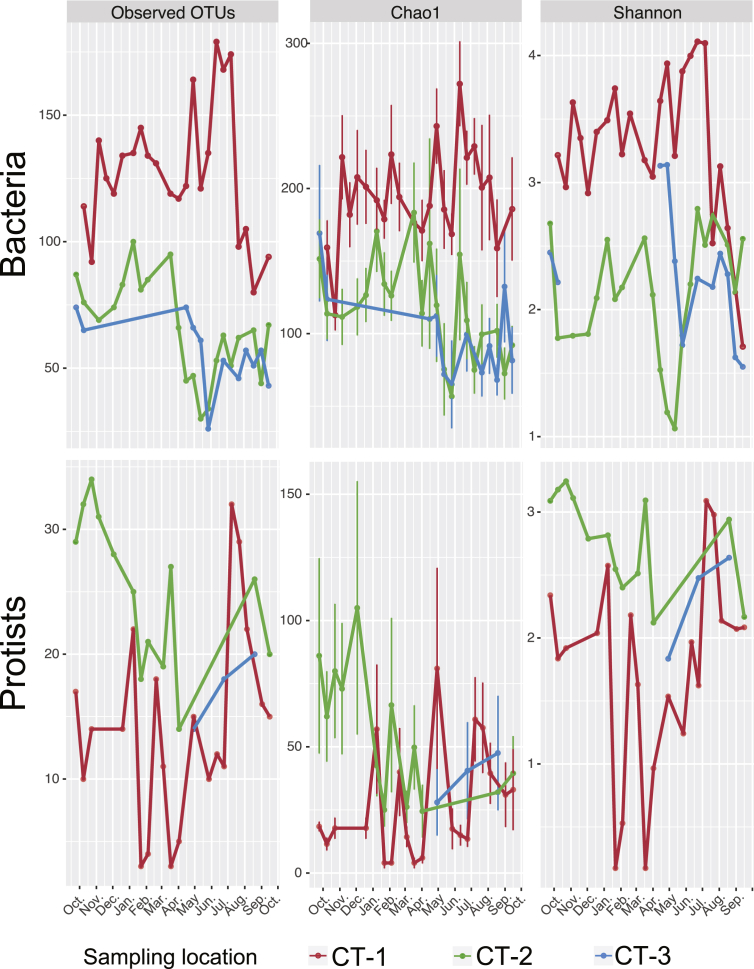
Bacterial and protist diversity in three cooling tower water basins. The number of observed OTUs and diversity indices for estimation of species richness and evenness of bacterial and protist communities are indicated for cooling towers CT-1, CT-2, and CT-3 over a sampling period of one year (September 2013 to September 2014). Pronounced differences between sampling sites and time points in both bacterial and protist diversity can be observed.
